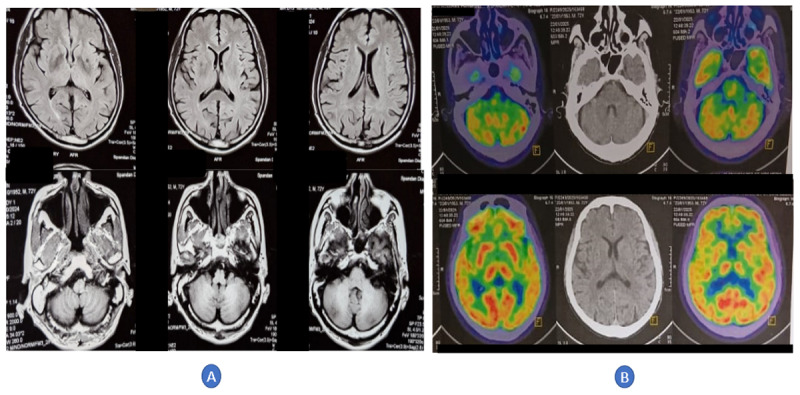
Structural MRI and 18F-FDG PET/CT findings. (A) Axial brain MRI shows a small chronic lacunar infarct in the left basal ganglia, minimal periventricular white-matter change, and age-appropriate cortical atrophy. (B) Brain 18F-FDG PET/CT shows mild asymmetric relative cerebellar hypometabolism, more evident in the left cerebellar hemisphere, with preserved uptake in the basal ganglia and cerebral cortex.
